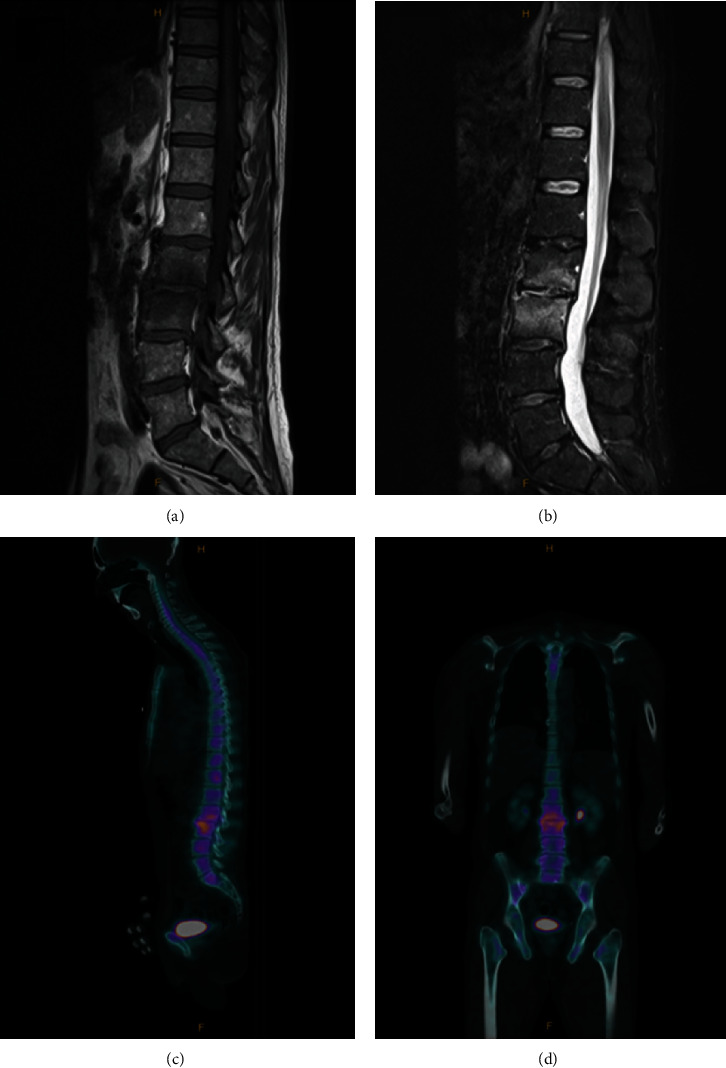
Magnetic resonance imaging (MRI) and single-photon emission computed tomography-computed tomography (SPECT-CT) images of the spine suggestive of L3/L4 discitis. Sagittal-view MRI whole spine demonstrates hypointense T1-weighted signal (a) and hyperintense short tau inversion recovery- (STIR-) weighted signal (b) surrounding the L3/L4 vertebral disc consistent with edema, with no evidence of collection. SPECT-CT whole spine shows increased uptake surrounding the L3/L4 vertebral disc in both sagittal view and coronal view consistent with L3/L4 discitis.
